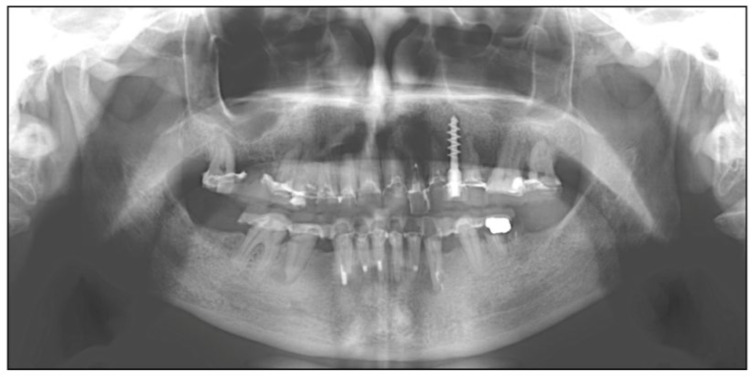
Preoperatory panoramic radiograph.
